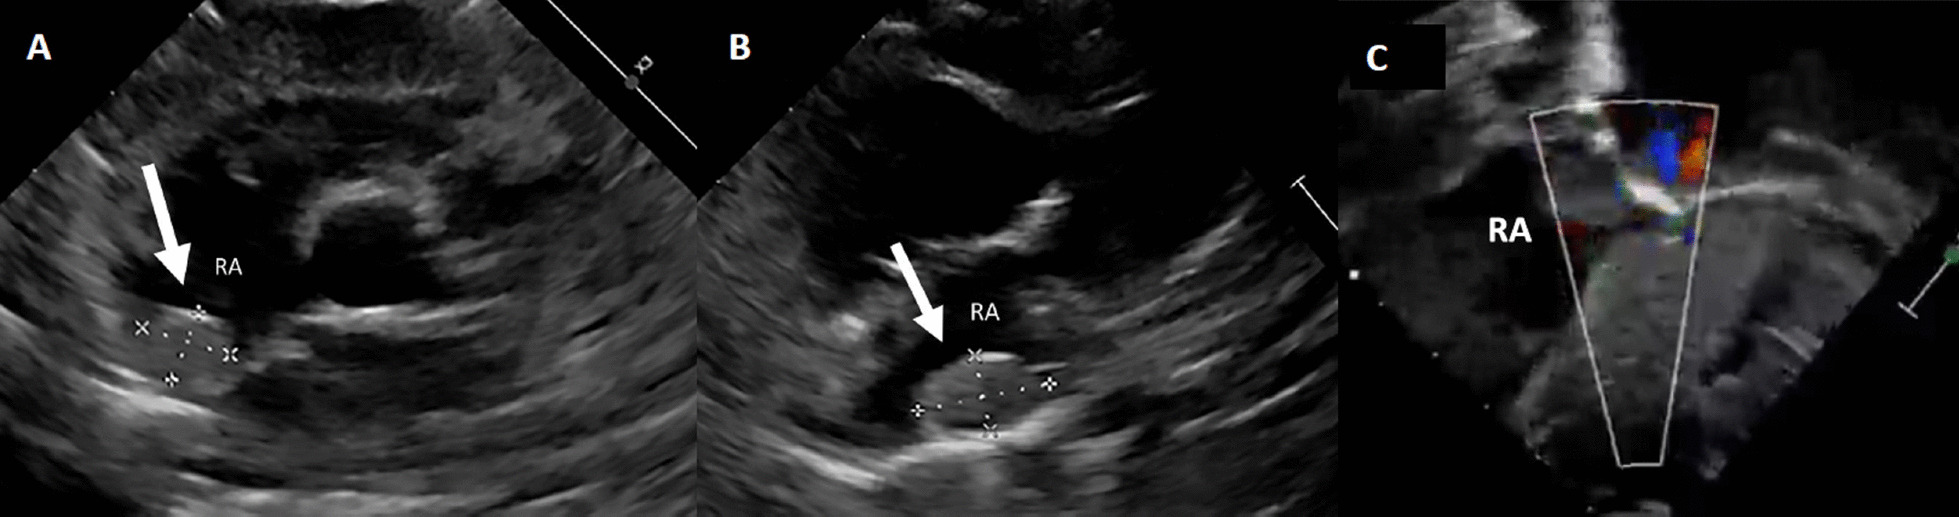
Case 11: Figure 3. TTE parasternal short-axis (A), high right parasternal (B), and subcostal sagittal (C) views. An intracavitary mass (arrow) is noted within the RA between the posterior RA wall and the interatrial septum. The mass is seen at the posterior RA wall, midway between SVC and IVC without systemic venous or tricuspid inflow obstruction. Doppler echocardiography showing lack of vascular flow signal of the RA mass
